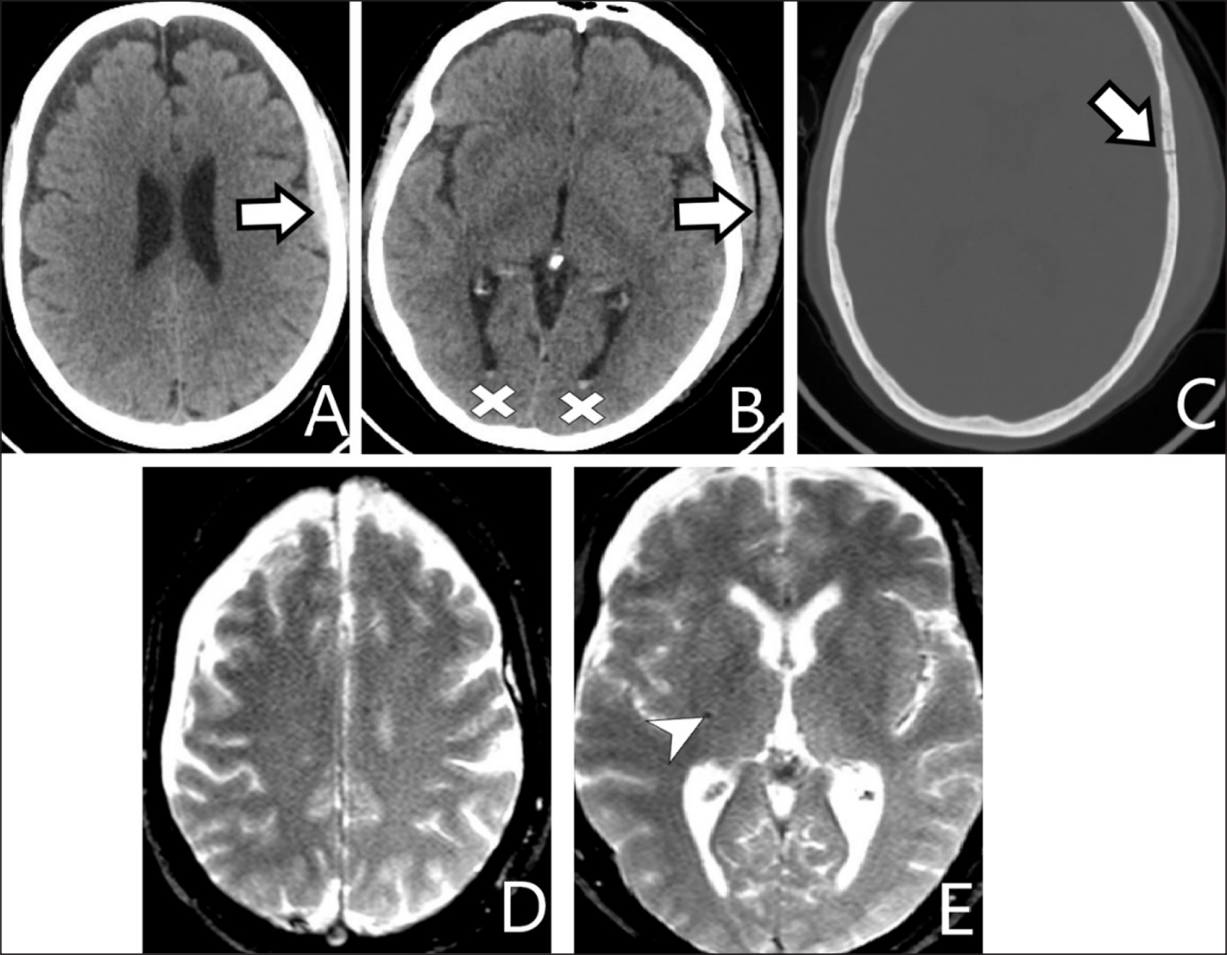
A *and* B: *axial soft-tissue window CT*, C: *bone window CT*, D *and* E: *T2*-WI*. Left frontal epidural hematoma and subgaleal hemorrhage with an underlying skull fracture (*arrows*). Small intraventricular bleedings in de occipital horns (*cross*). Diffuse petechial bleeds, one particular focal hemorrhage in the posterior limb of the right internal capsule (*arrowhead*).
